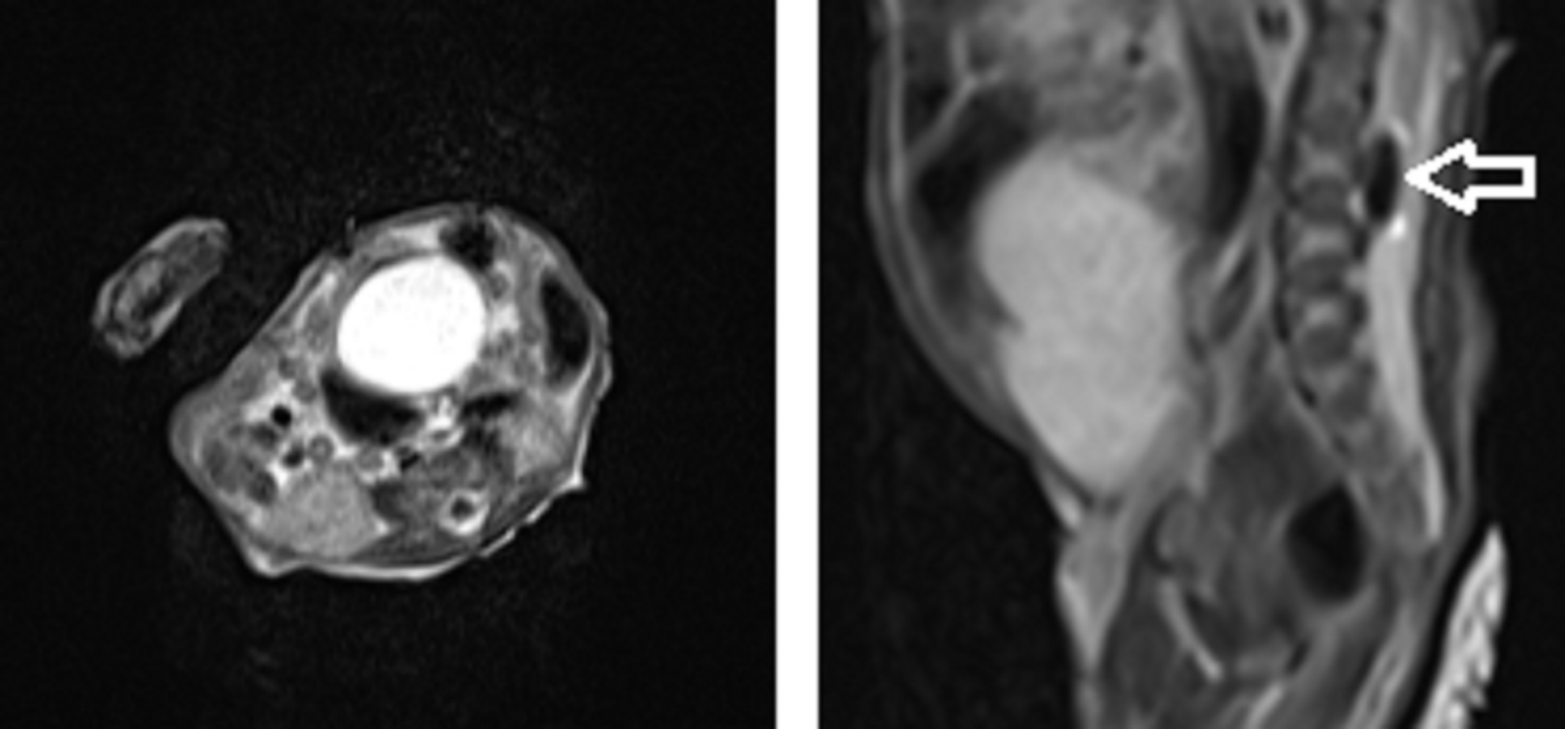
MRI of Lumbar Spine Sagittal T2 (Right) and axial T2 (Left) sequence, respectively, demonstrating a hypointense lesion in the anterior thecal sac extending from L2-L3 consistent with a large air bubble (Arrow)
